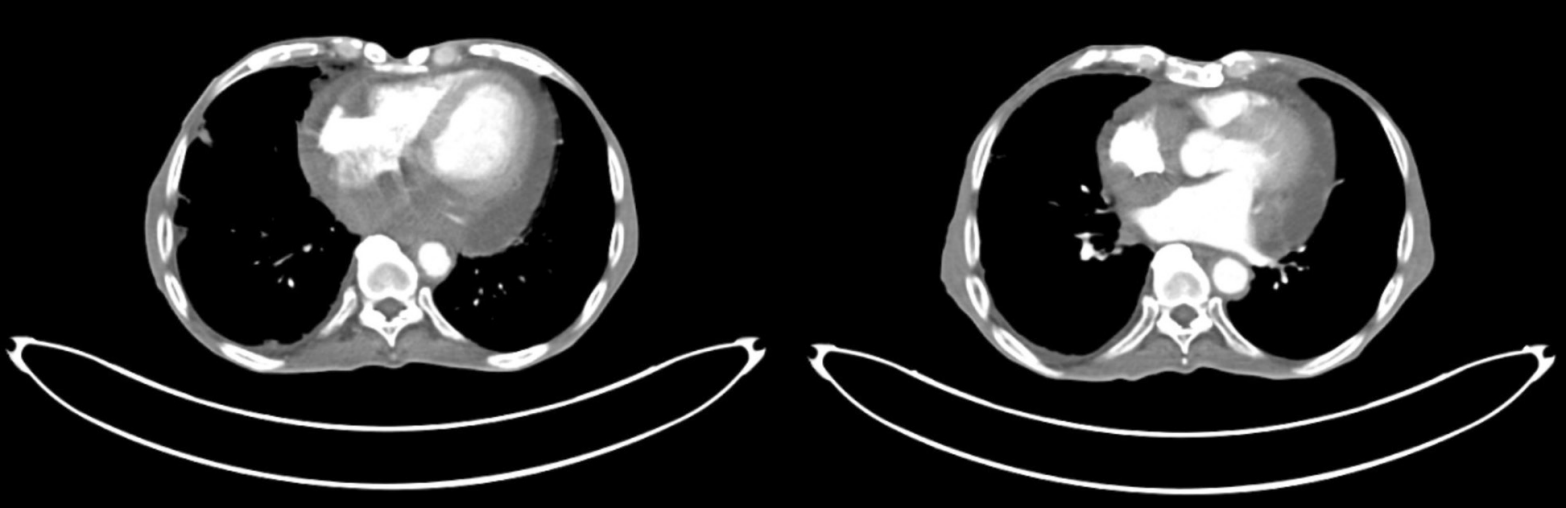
Contrast‐enhanced computed tomography (CECT) showing paracardiac soft tissue enhancement surrounding both the atria, basal parts of ventricles, atrio‐ventricular grooves and medial aspect of ascending aorta.
